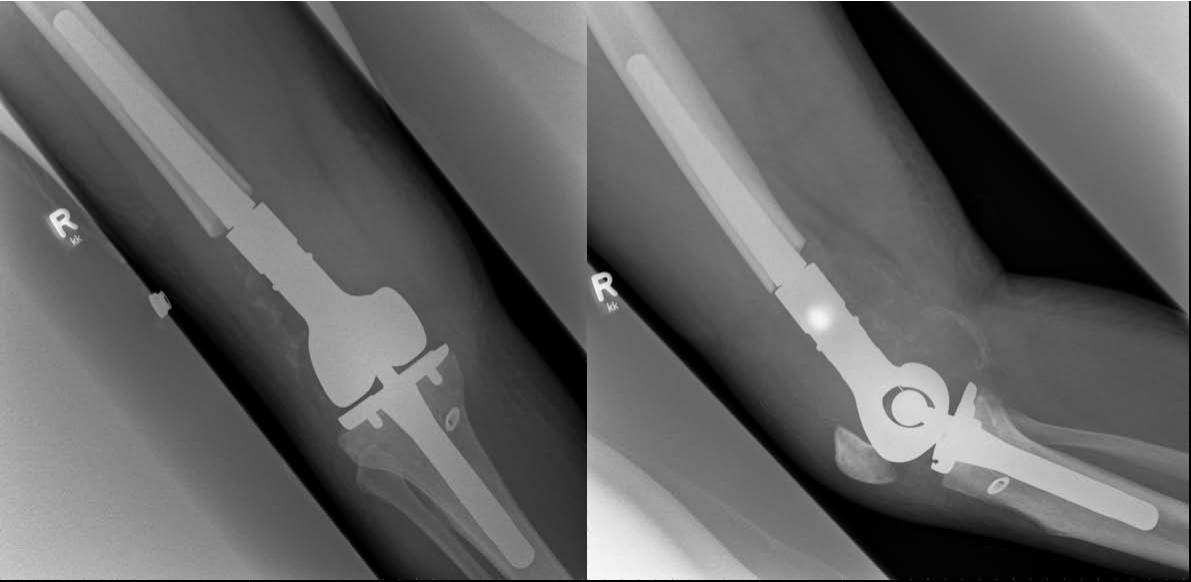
Conventional X-ray imaging in anteroposterior (a) and lateral (b) projection after the implantation of a silver-coated non-cemented modular knee joint prosthesis (MUTARS).
